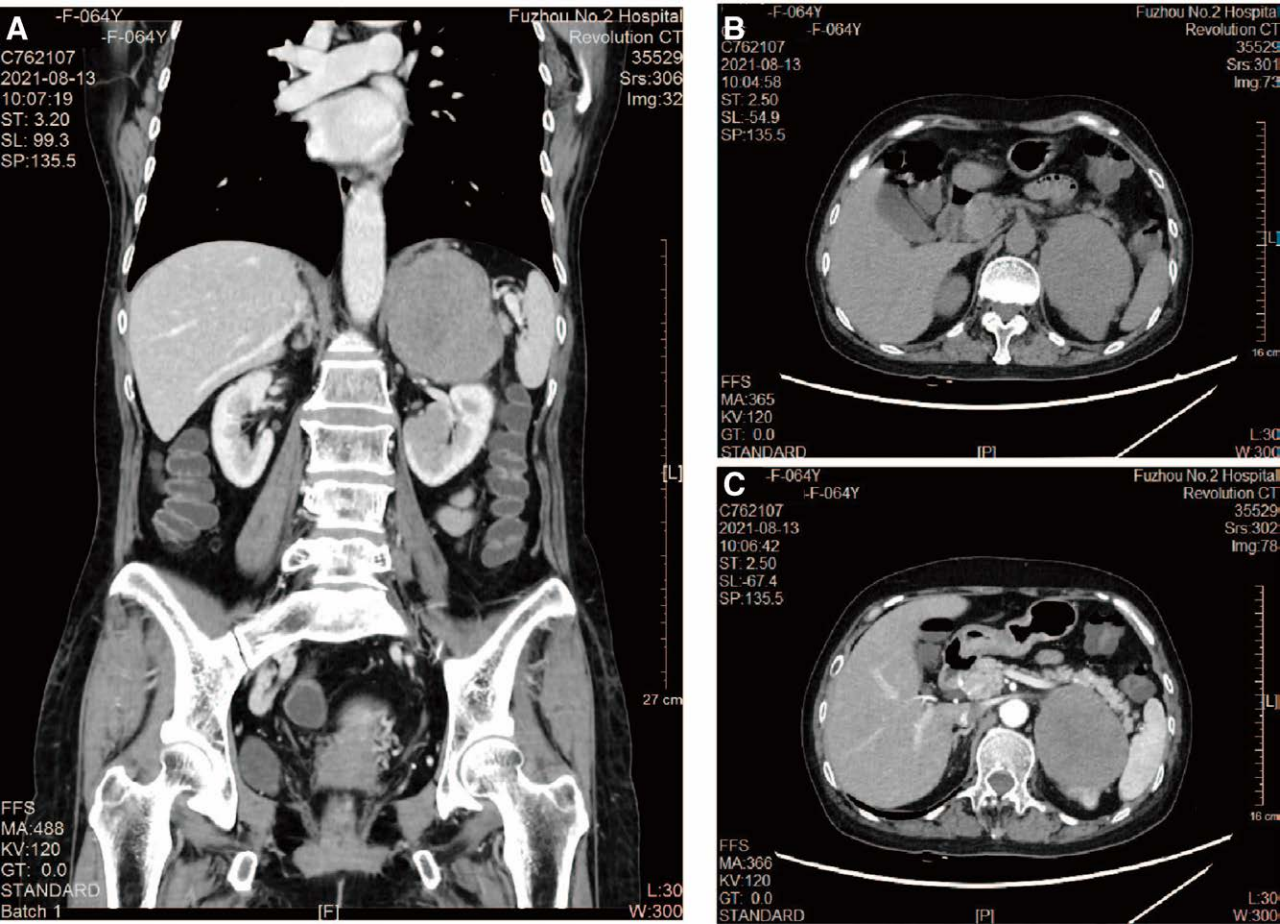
Computed tomography of the whole abdomen. A. Coronal enhancement; B. Cross-sectional plain scan; C. Cross-sectional enhancement.
